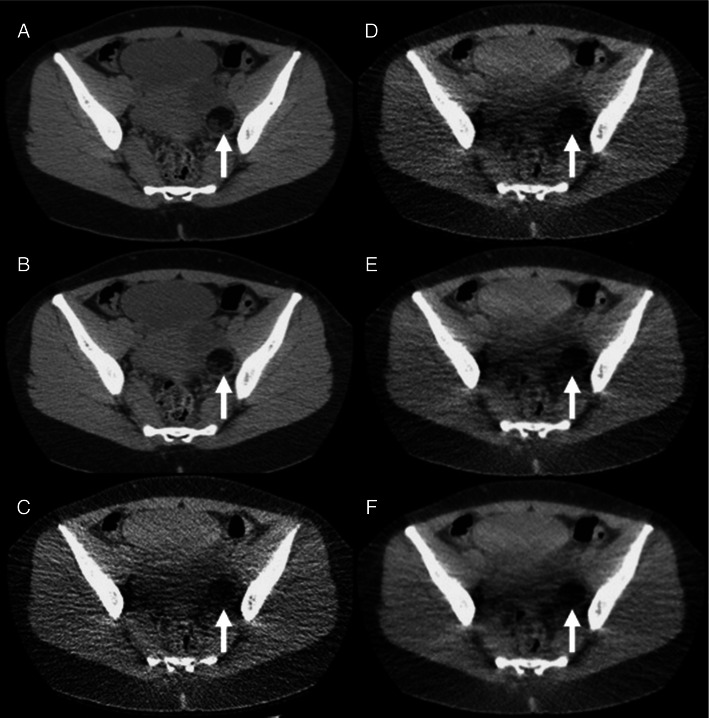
A 29-year-old female patient (BMI = 20.8) with haematuria. a and b Axial conventional dose (3.6-mSv) 5-mm non-contrast CT images reconstructed with 40 % ASiR (a) and 100 % FBP (b) showing a 5.4-cm low-density left adnexal mass consistent with a dermoid cyst (*arrow*). This left adnexal mass was missed when viewed on the low-dose non-contrast CT images. c-f Axial low-dose (0.48-mSv) 5-mm non-contrast CT images reconstructed with 100 % FBP (c), 40 % ASiR (d), 70 % ASiR (e) and 90 % ASiR (f) showing the inferior detail of the left adnexal lesion relative to the conventional dose images (*arrow*). Again note the ‘over smoothening’ of the soft tissues on the 90 % ASiR image compared to the other low-dose reconstructions
